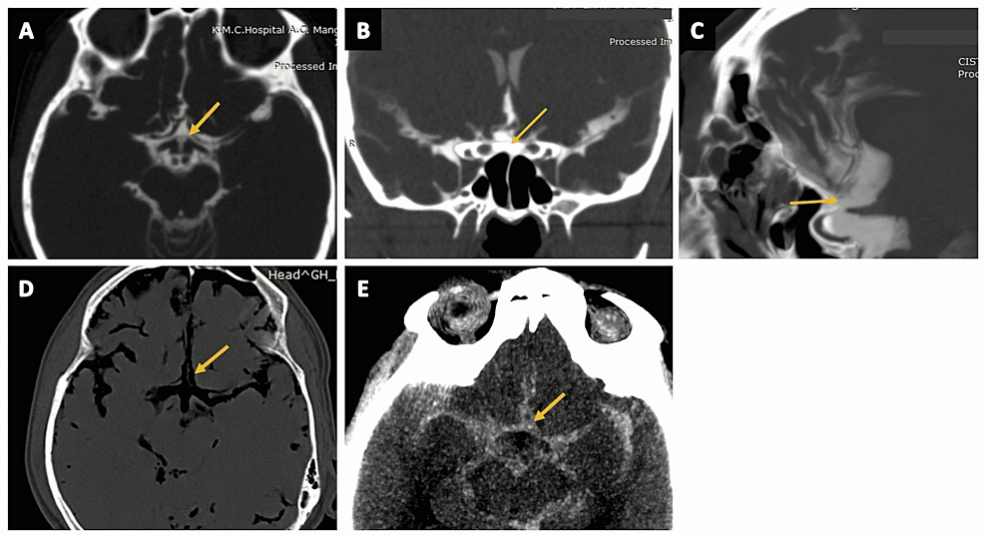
The cisterna laminae terminalis (cistern of lamina terminalis). (A), (B), and (C) show the cistern of lamina terminalis (arrows) as seen on the axial, coronal, and sagittal sections of CT cisternography, respectively, in a case of suspected CSF rhinorrhea. (D) The cistern of lamina terminalis (arrow) as seen on the axial section of CT brain (negative contrast) in a case of pneumocephalus. (E) The cistern of lamina terminalis (arrow) as visualized on the axial plane of CT brain (positive contrast) in a case of subarachnoid hemorrhage (SAH).
